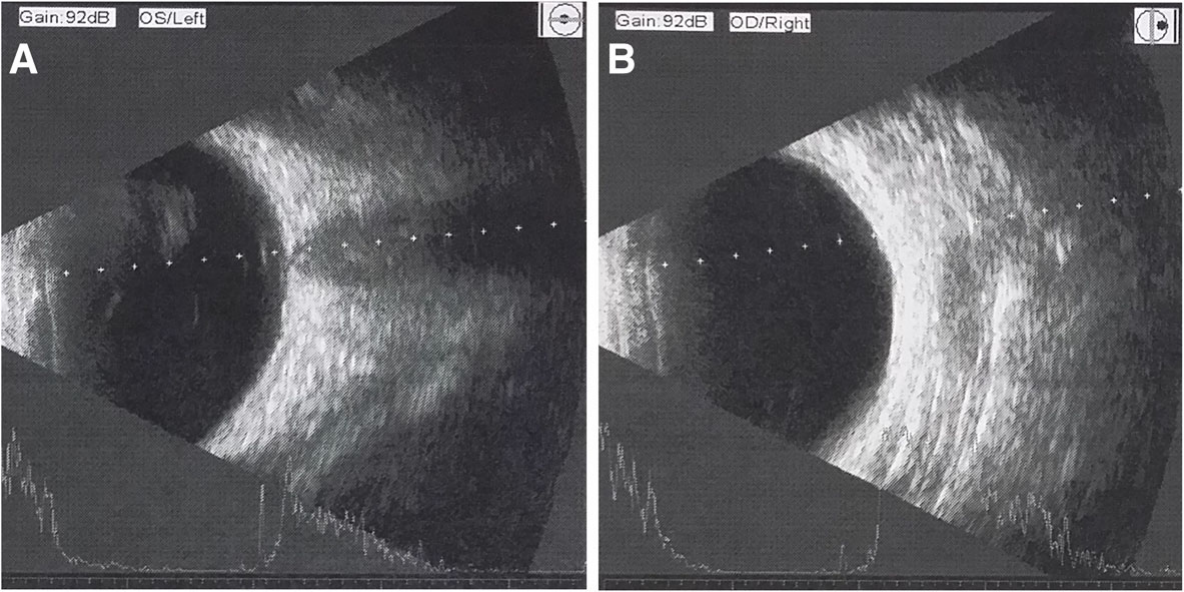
Ultrasound B-scan of the posterior segment in the left eye (a) and the fellow eye (b)
